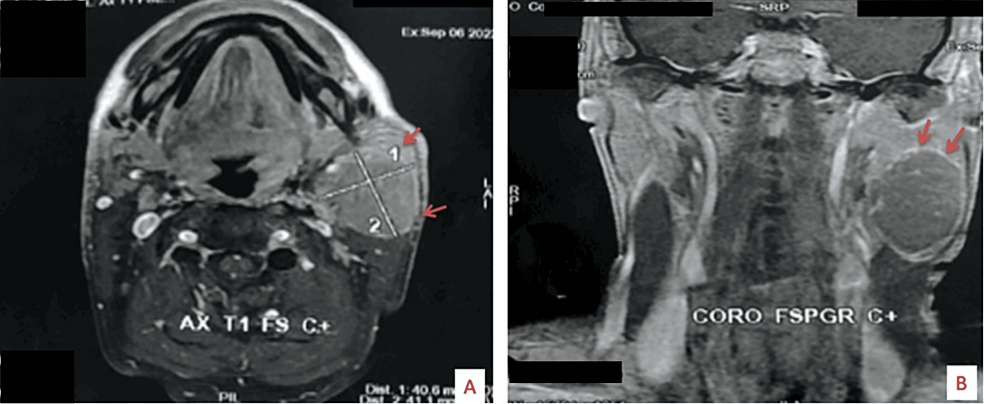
MRI coronal (A) and axial (B) sections showing a 6 cm synovial sarcoma of the left submaxillary gland (red arrows).
